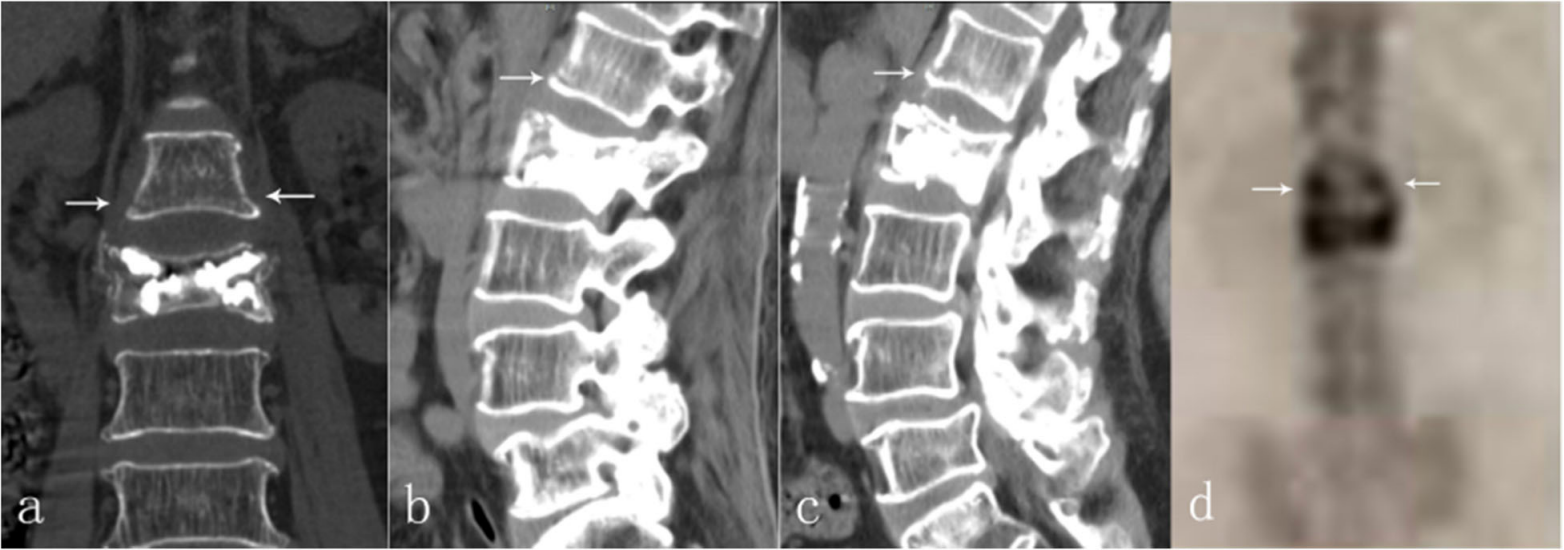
CT examination 2 weeks after L2-PVP detected an ambiguous fracture line at L1 (a-c, arrow), later reconfirmed by bone scintigraphy as fresh vertebral fracture (d, arrow)
